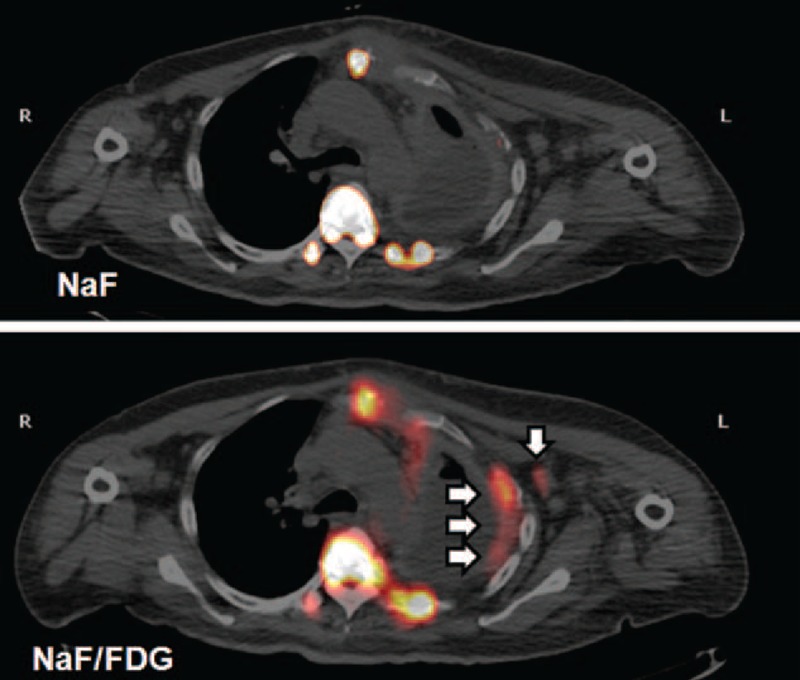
A cocktail of F-18 NaF/FDG revealed soft tissue uptake in left axillary nodes and left pleura (white arrows) and surpraclavicular nodes. Surgical biopsy was performed in the left axillary nodes 2 days later according to these findings. Pathology revealed poorly differentiated adenocarcinoma of breast origin. FDG = fluorodeoxyglucose, NaF = sodium fluoride.
